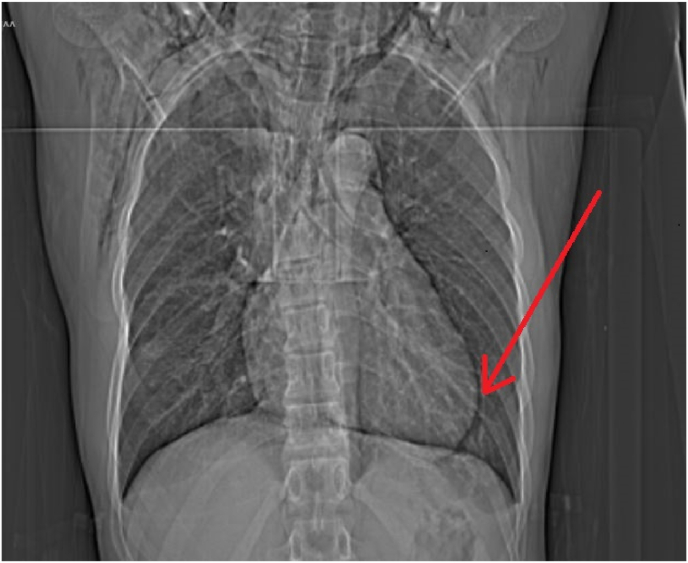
CT Scout View showing an air around the heart i.e pneumopericardium (arrow).
